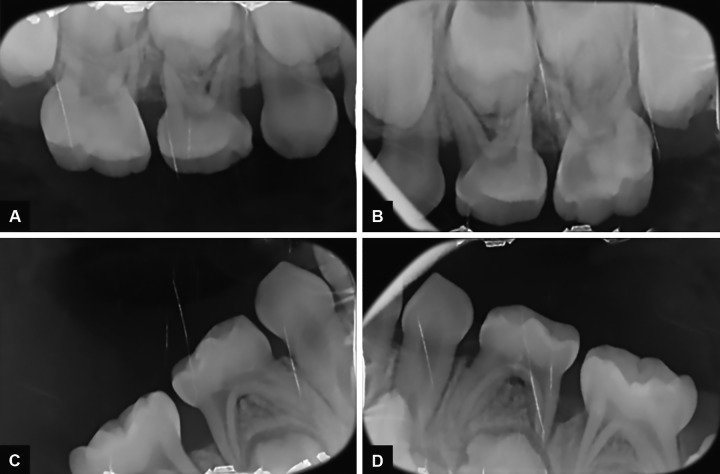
Initial radiographic examination of the patient
